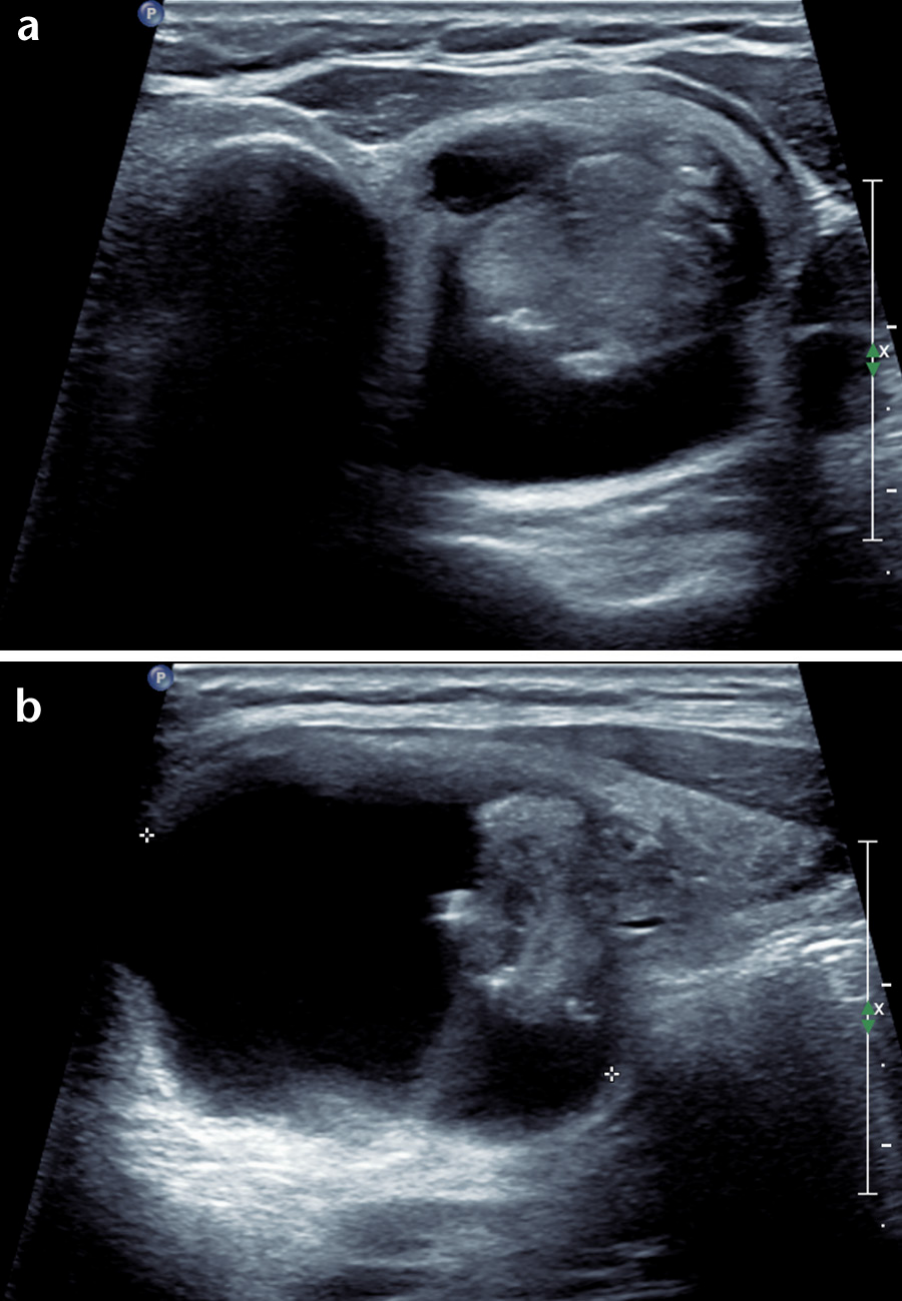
Axial (a) and longitudinal (b) ultrasound of a papillary carcinoma in the left lobe: a well-defined mixed composition nodule with isoechoic solid component however the solid focus is eccentrically positioned forming a mural nodule that has an acute angle with the cyst wall.
